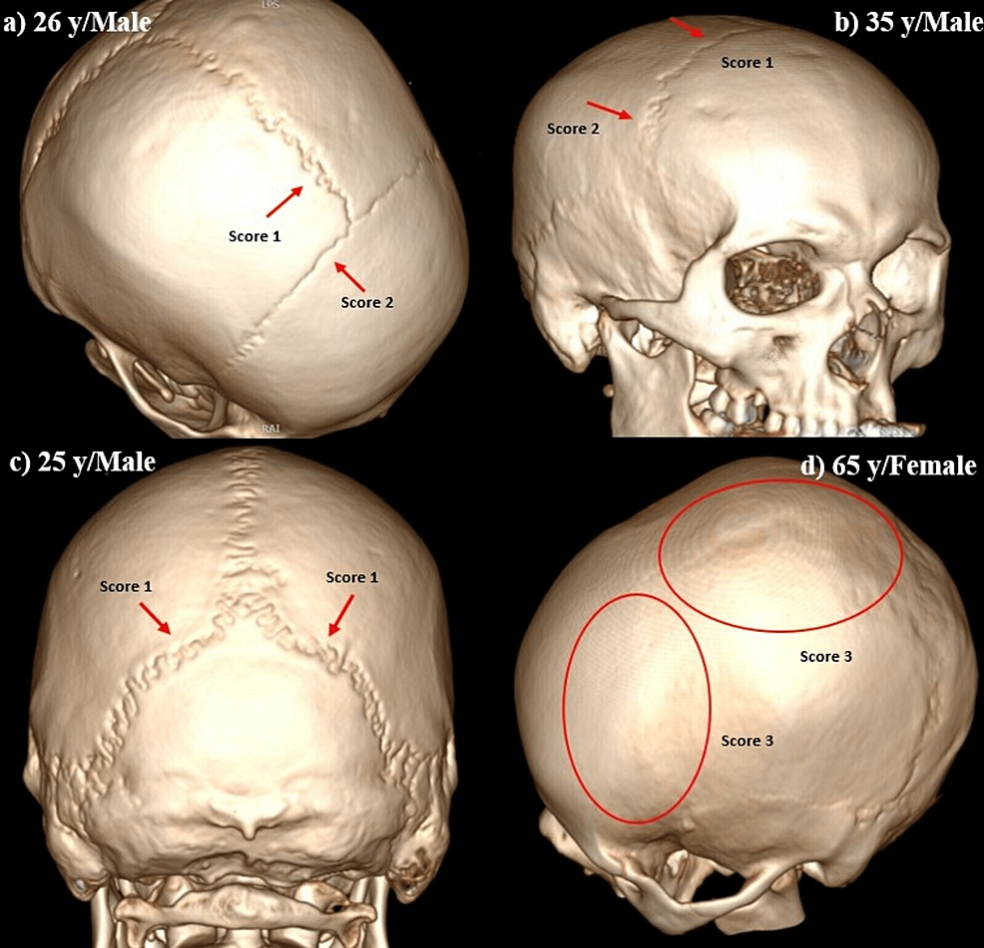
Three-dimensional CT image of skull indicating suture closure a) Score 1 of the sagittal suture and score 2 of the coronal suture, b) scores 1 and 2 in the coronal suture on right side, c) score 1 on the lambdoid suture, and d) score 3 on the coronal and sagittal suture. The red arrows indicate the cranial suture closure of scores 1 and 2, and the red circles indicate the complete closure of the suture with a score of 3.
